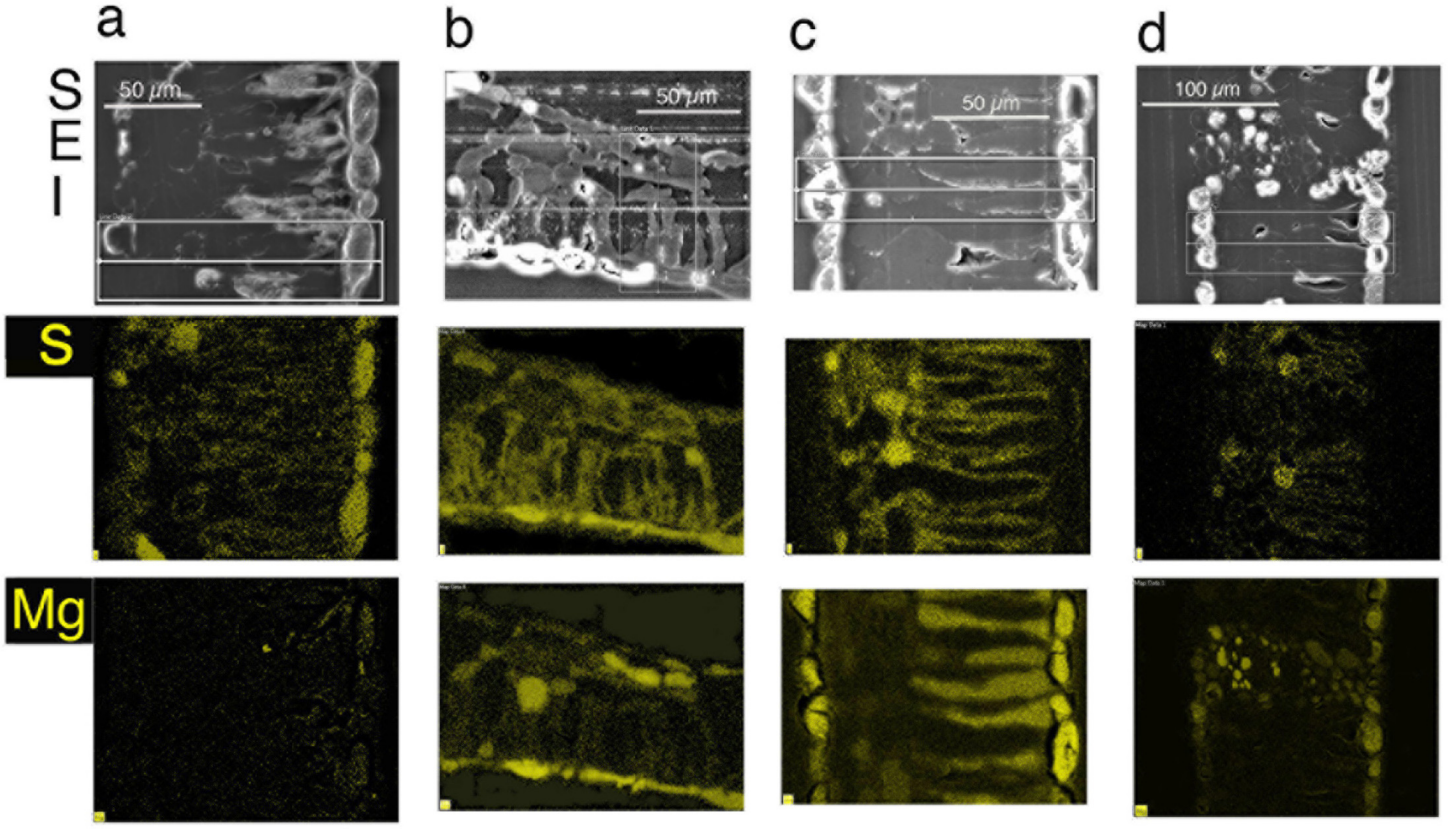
Secondary electron images (SEI) in the top horizontal panel (same sample as in Figs 2 and 3), with corresponding colour-coded X-ray maps directly below, showin *in vivo* S, and Mg maps in the two panels below.
